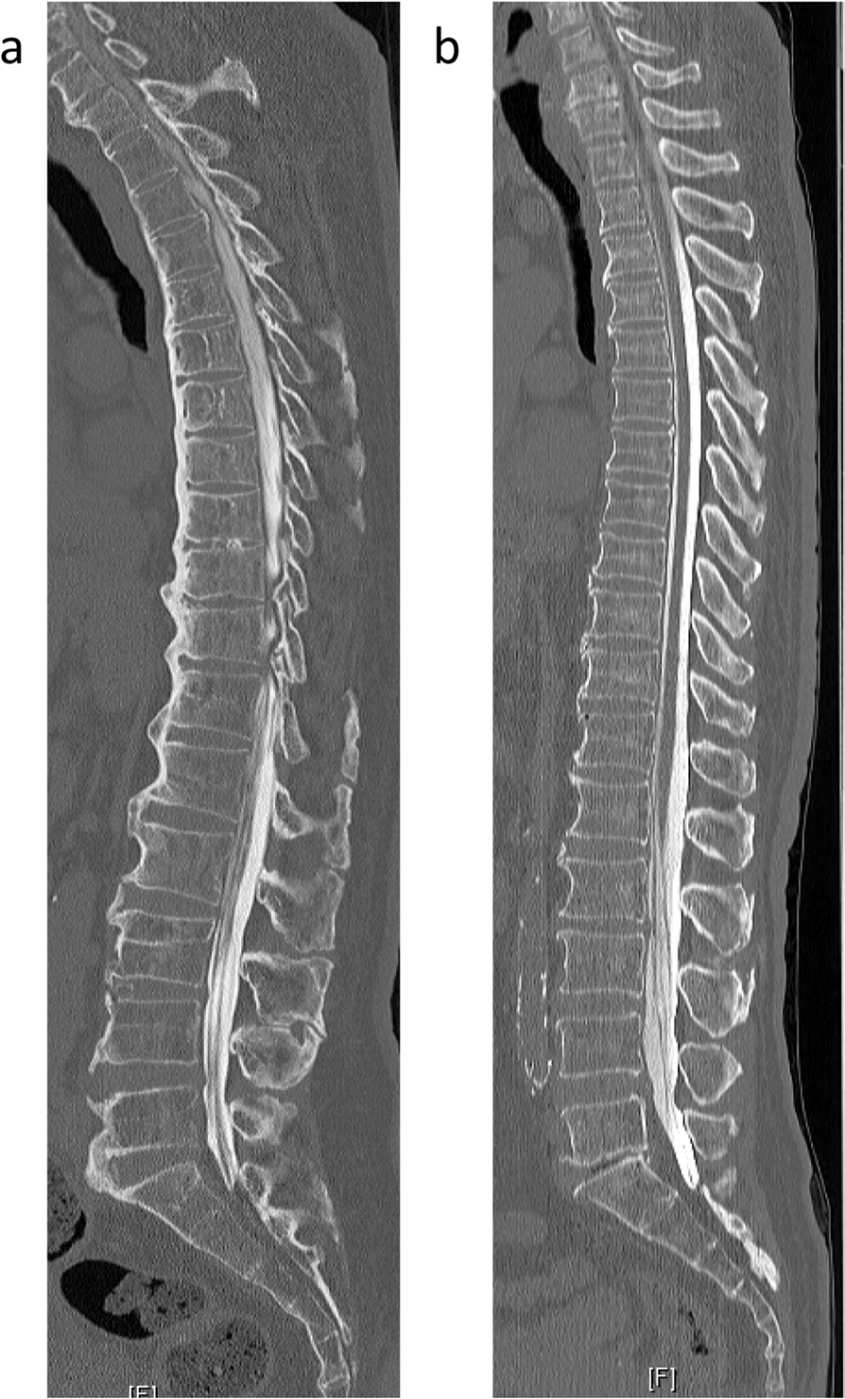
Reconstructed sagittal computed tomography images. a Patient with DISH. b Patient without DISH. DISH, diffuse idiopathic skeletal hyperostosis
